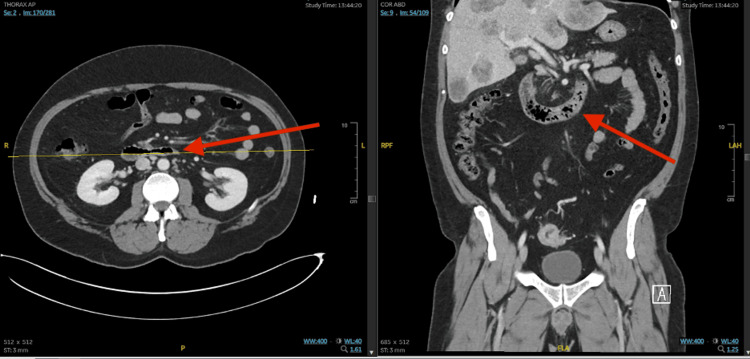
Axial and coronal abdominal CT scans on the day of presentation The CT scans show significant thickening of the small bowel wall in the jejunum.
